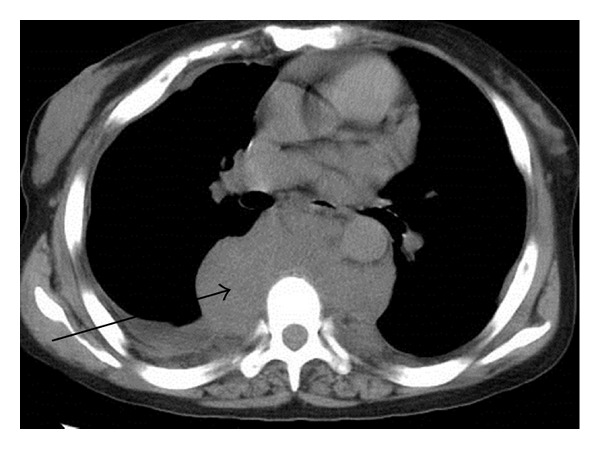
Post-treatment CT result suggests bilateral pleural effusions were largely not evident, however soft masses surrounding the vertebrae still exist. On March, 6, 2011, the CT scan was performed following treatment. The left arrow indicated a rounded soft tissue mass surrounding the vertebrae.
